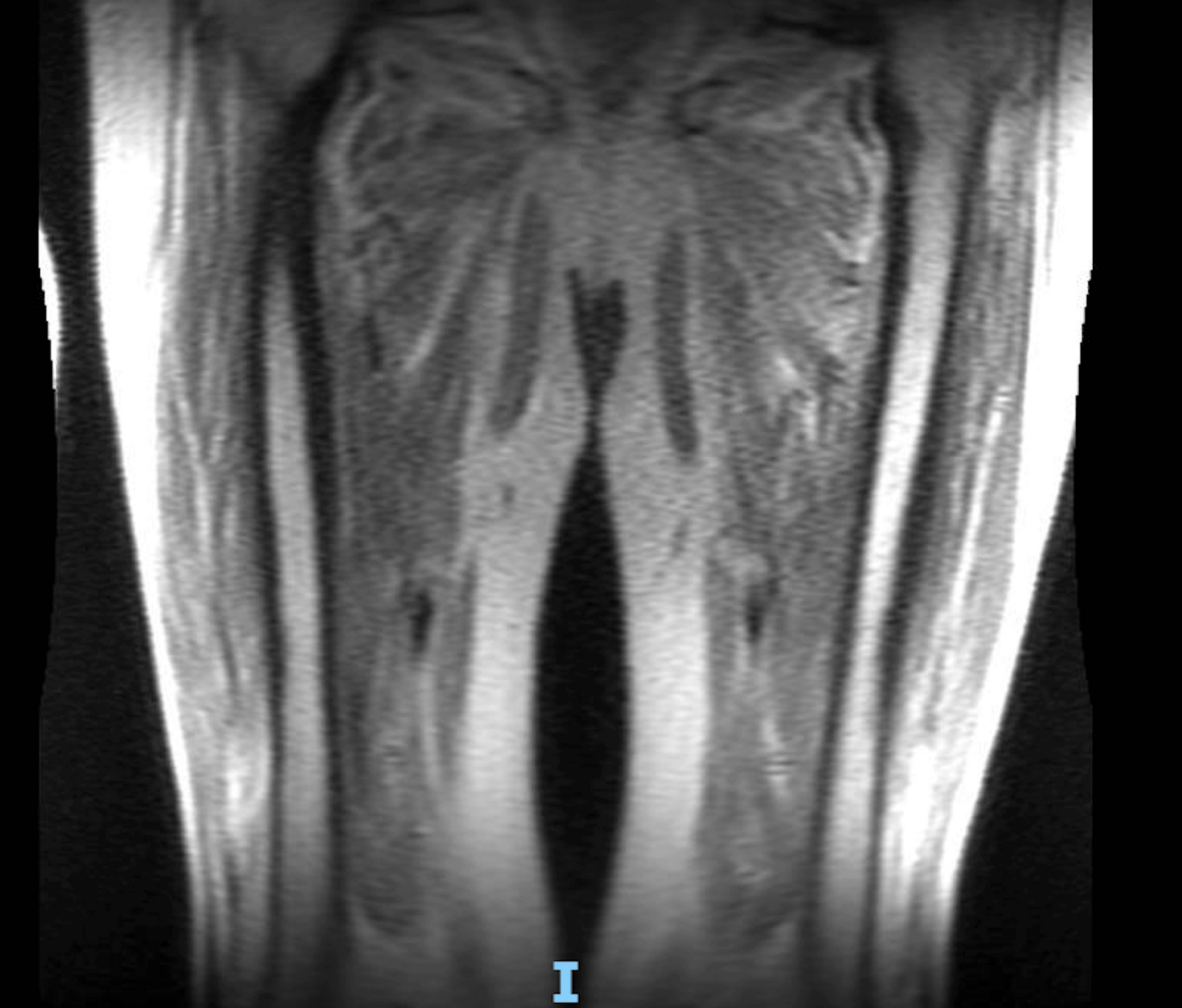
MRI of the femur demonstrating extensive muscle edema in both the thighs
